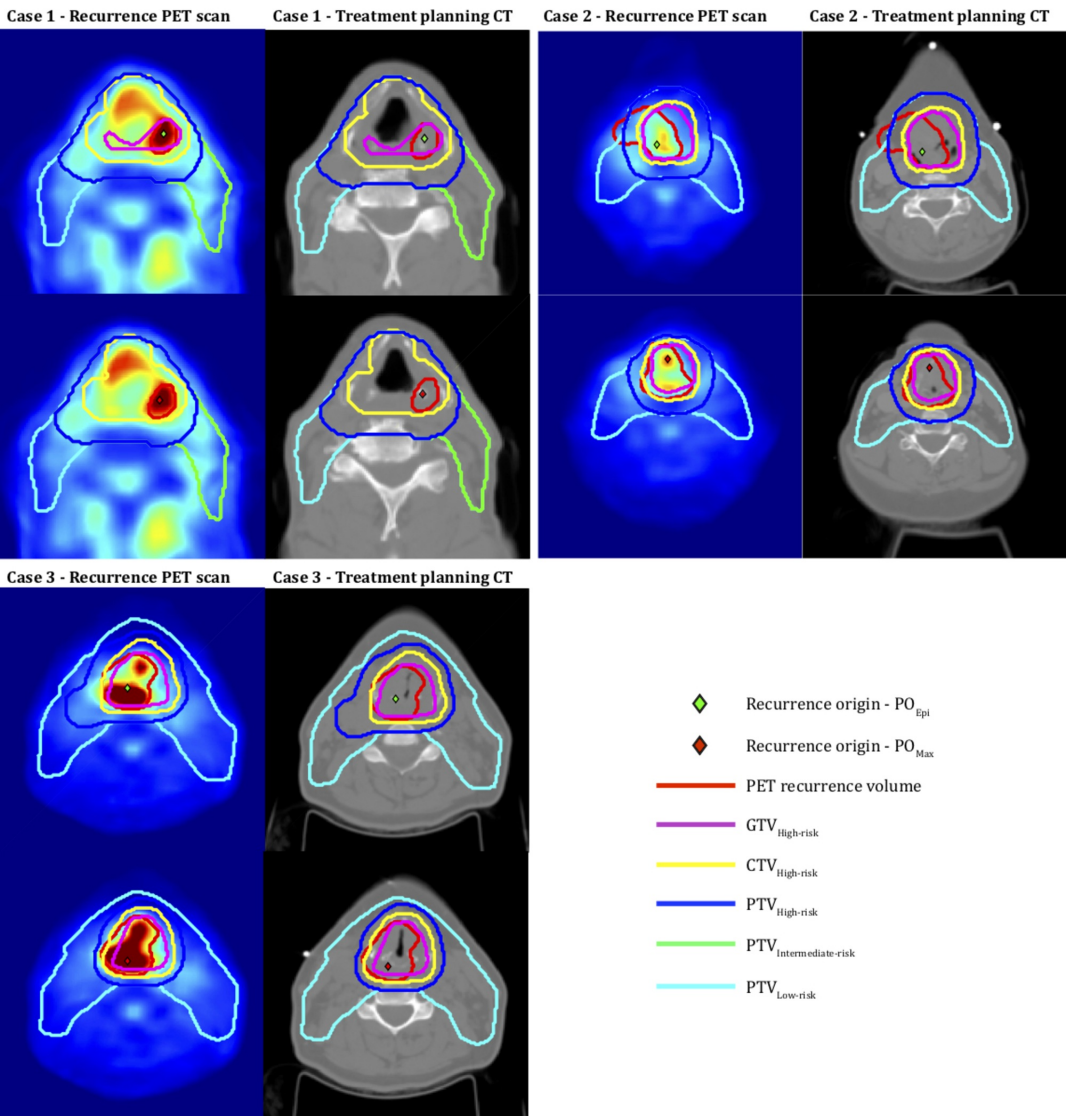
Recurrence origin points determined by the two methods for three of the patients in this analysis Calculated recurrence origin points and target volumes are shown overlayed on a cross-sectional view of the treatment planning CTs and follow-up PET scans, respectively. If the corresponding recurrence origin point falls within a given target volume, it is scored as having originated from within this and any larger target volumes encompassing that one. CT: computed tomography; CTV_High-risk_: high-risk clinical target volume; GTV_High-risk_: high-risk gross tumor volume; PET: positron emission tomography; PO_Epi_: mathematical epicenter point of origin; PO_Max_: maximum standardized uptake value point of origin; PTV_High-risk_: high-risk planning target volume; PTV_Intermediate-risk_: intermediate-risk planning target volume; PTV_Low-risk_: low-risk planning target volume
